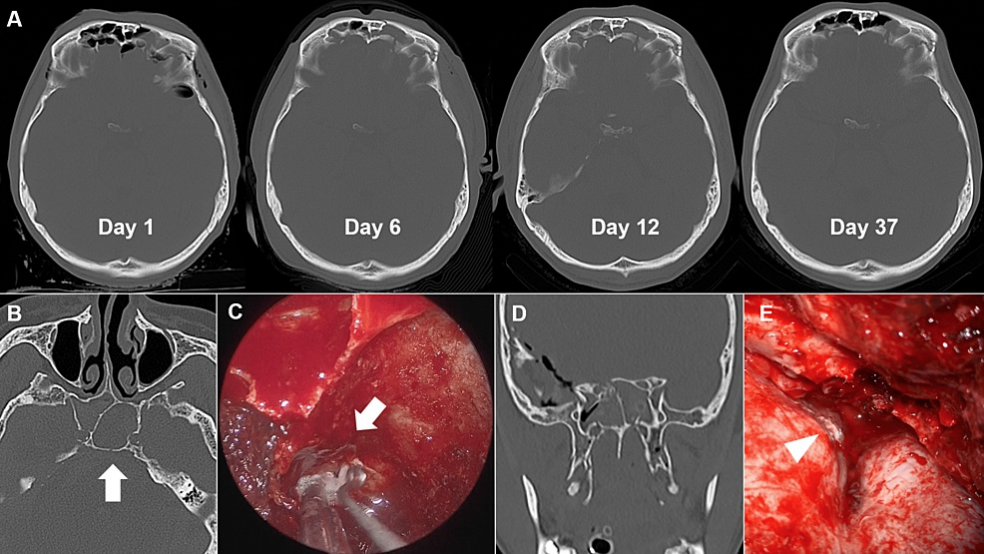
Imaging findings (A) Computed tomography (CT) scan of the brain revealing changes in air content in the frontal sinus over time, (B) CT scan showing a fracture of the clivus (arrow), (C) an intraoperative view of endoscopy showing fat filling in the cerebrospinal fluid (CSF) leak site of the clivus (arrow), (D) CT coronal view showing compound fractures of the anterior skull base, and (E) intraoperative view showing a site of dural injury in the anterior skull base leaking CSF (arrowhead).
